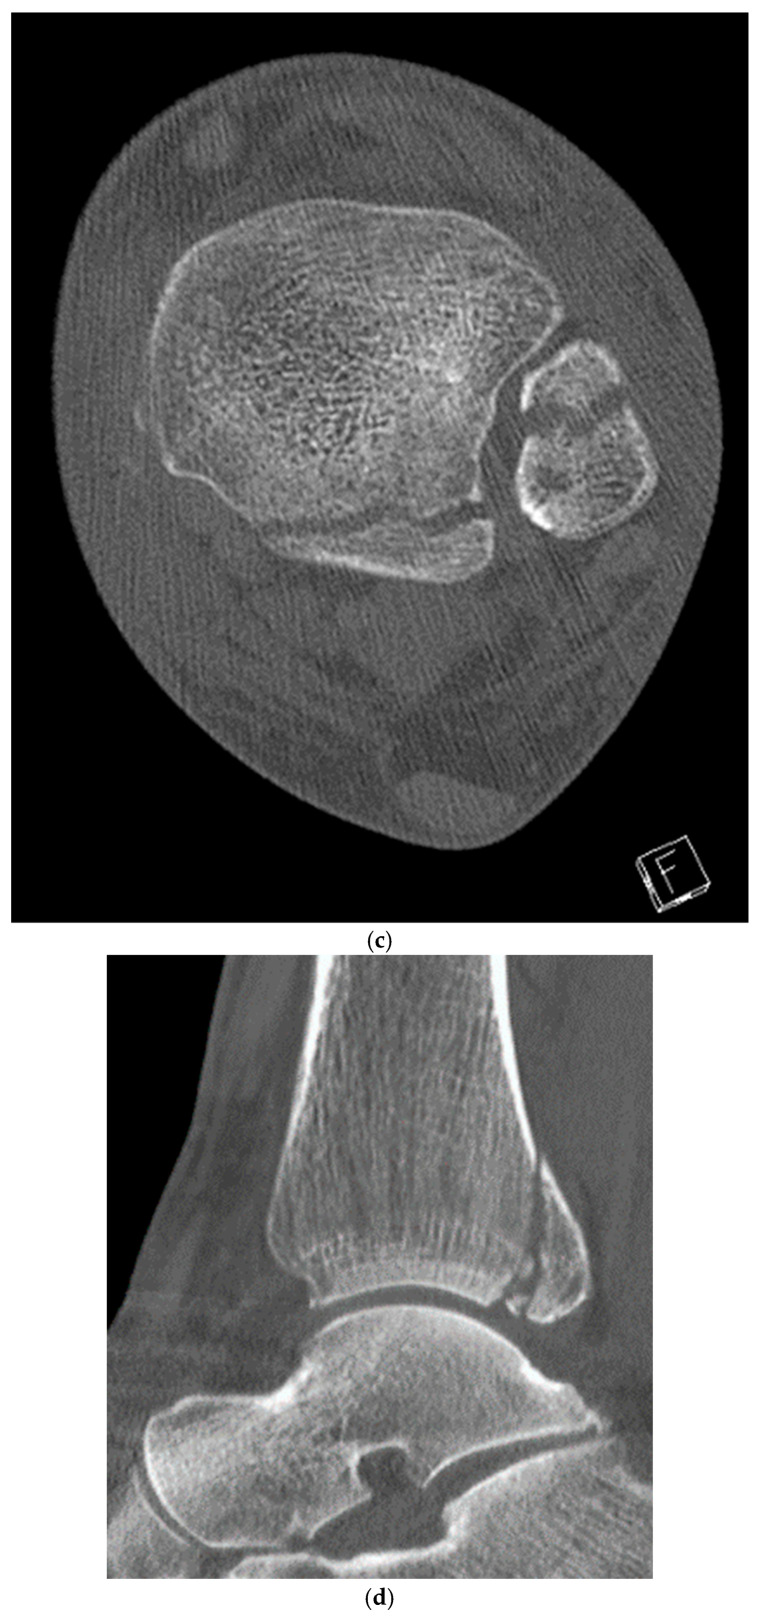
(a–d): Trimalleolar ankle fracture: (a) anteroposterior and (b) lateral radiographs; (c) axial and (d) sagittal CT views demonstrating type 2 posterior malleolar fracture with small intercalary fragments.
